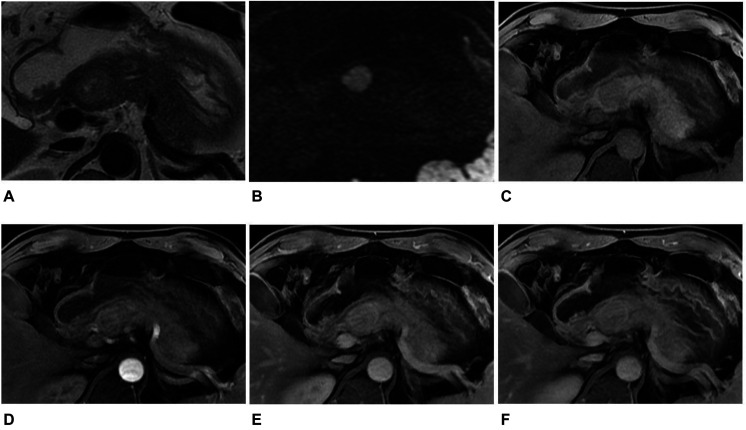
MR images in a 45-year-old man with a non-hypervascular PNET. (A) Axial T2-weighted image showed a well-circumscribed high signal intensity tumor in the head of the pancreas. (B) The tumor appeared as high signal in DWI with *b* = 1,500 s/mm^2^. (C–F) Axial T1-weighted images obtained during plain (C), arterial (D), portal venous (E), and delayed (F) phases. The tumors showed enhancement in the arterial, portal venous, and delayed phases when compared with the adjacent parenchyma. The diagnosis of pre-operation MRI was SPT. However, the radiomics analysis showed that the tumor was PNET.
